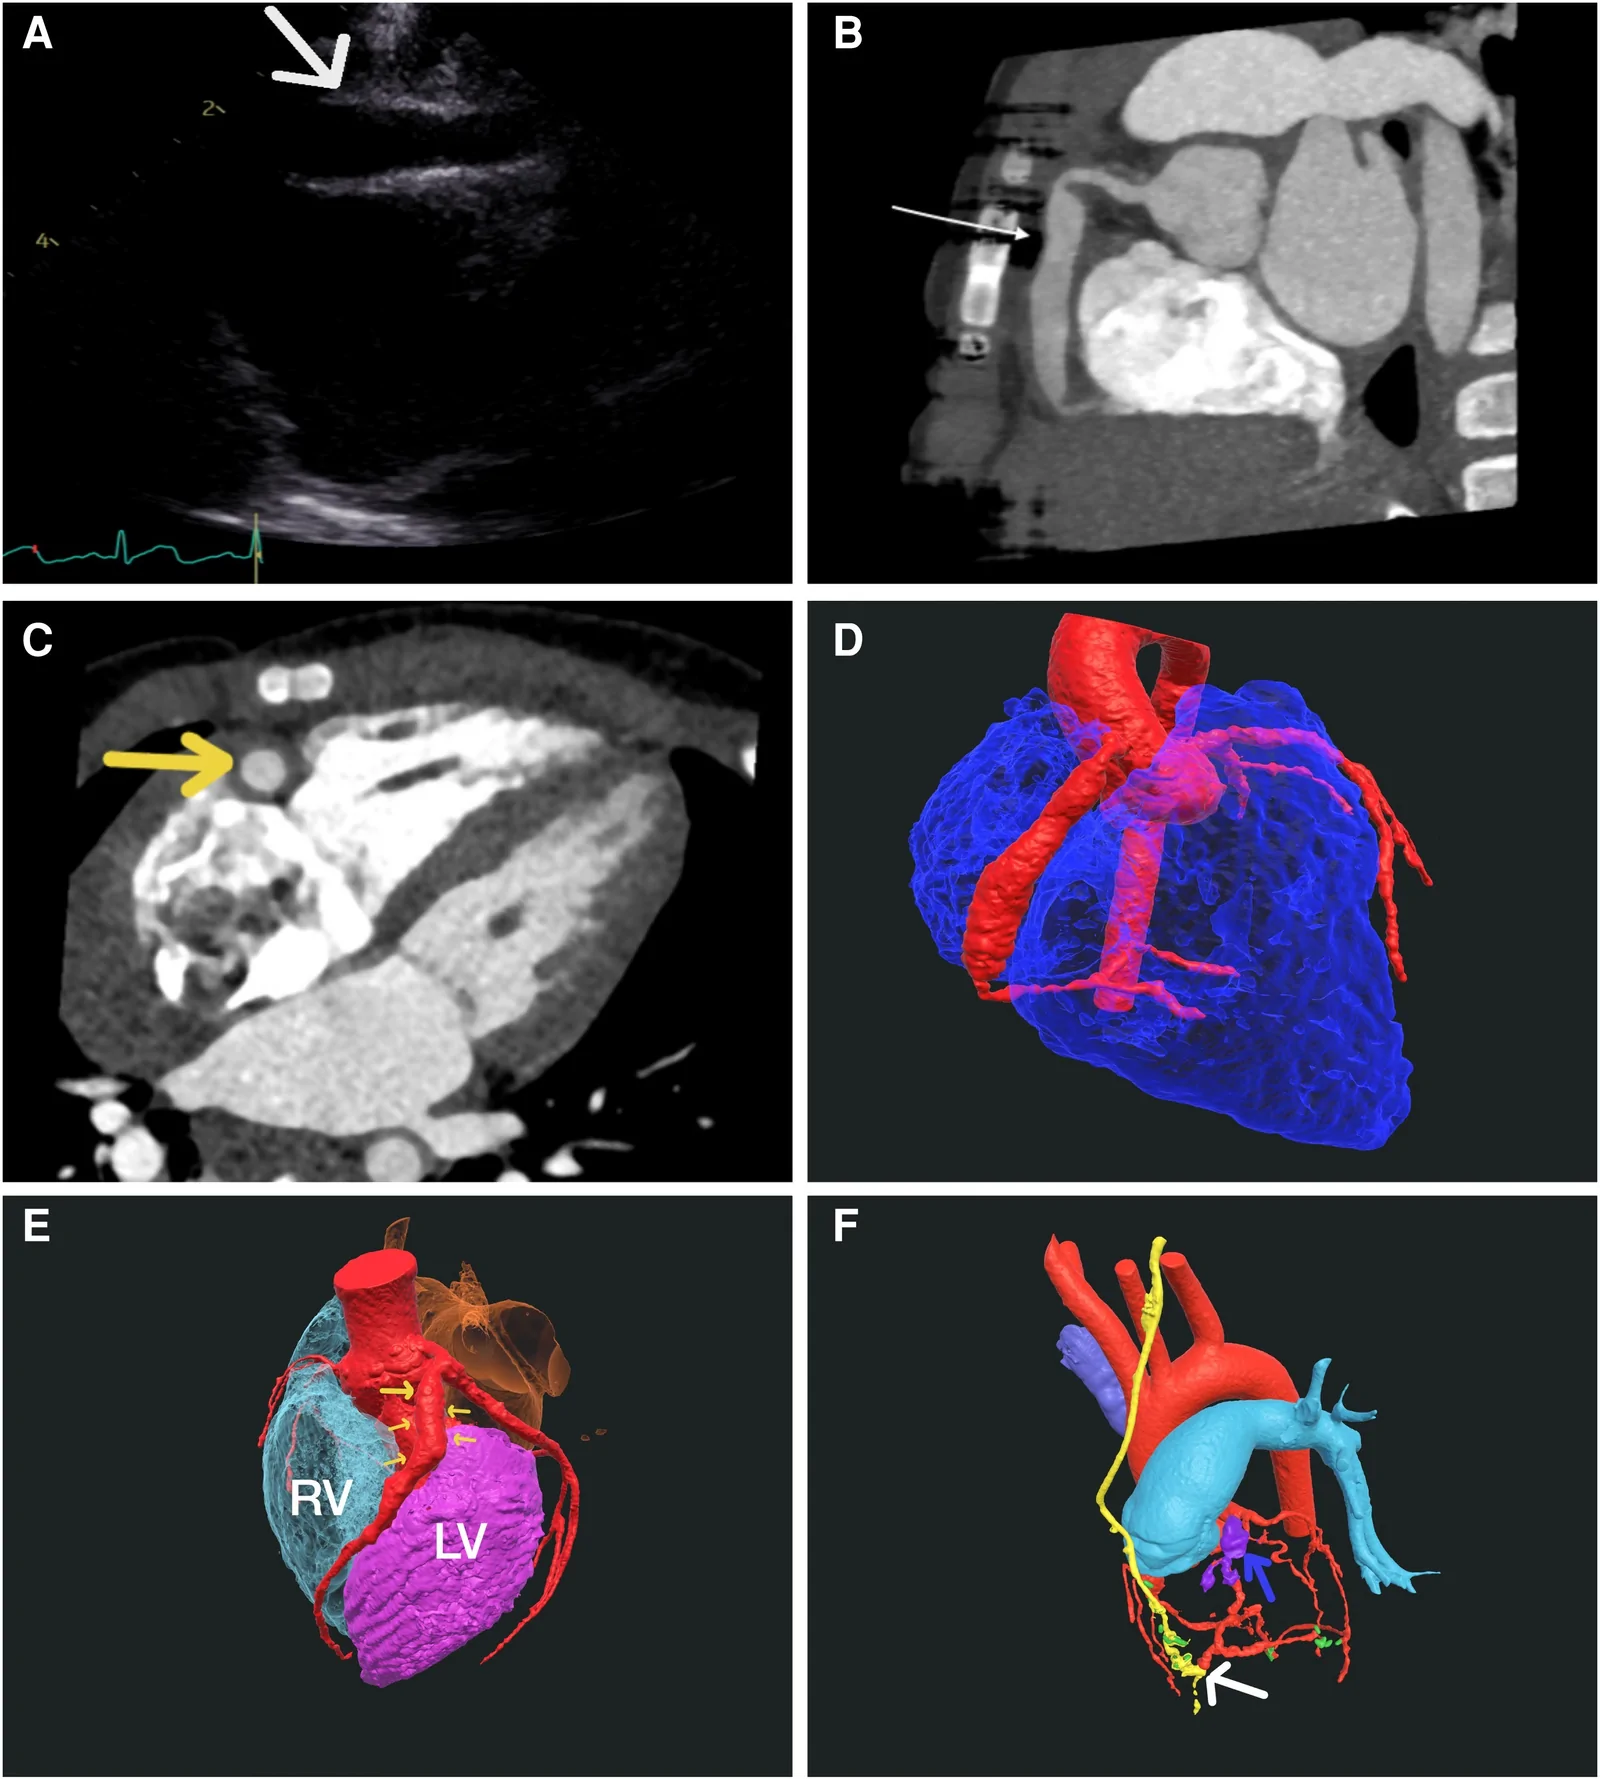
Mapping the coronary aneurysm landscape in Kawasaki disease. An 8-month-old boy with Kawasaki disease shown in panels *A–D*. (*A*) Transthoracic echocardiography shows a giant right coronary artery (RCA) aneurysm (solid white arrow). (*B*, *C*) Computed tomography (CT) angiogram demonstrates the full longitudinal extent of the giant RCA aneurysm (white arrow and yellow arrows). (*D*) Virtual reality (VR) rendering depicts the aneurysm with improved spatial and anatomical appreciation, the RCA (red) is positioned along the right atrioventricular groove. The right atrium (RA) and right ventricle (RV) are shown in transparent blue. (*E*) VR rendering of a 16-year-old girl with Kawasaki disease showing a large left anterior descending (LAD) coronary artery aneurysm (yellow arrows). The aorta and coronary arteries are shown in red; the RV in transparent blue; and the left ventricle (LV) in light purple. (*F*) VR rendering of a 14-year-old boy with Kawasaki disease demonstrates a thrombosed LAD aneurysm (purple; blue arrow), and a left internal mammary artery (LIMA) graft (yellow). The graft anastomosis with the distal LAD is indicated by the white arrow. The aorta, aortic branches and coronaries are shown in red, the pulmonary arteries in light blue; superior vena cava (SVC) in light purple.
